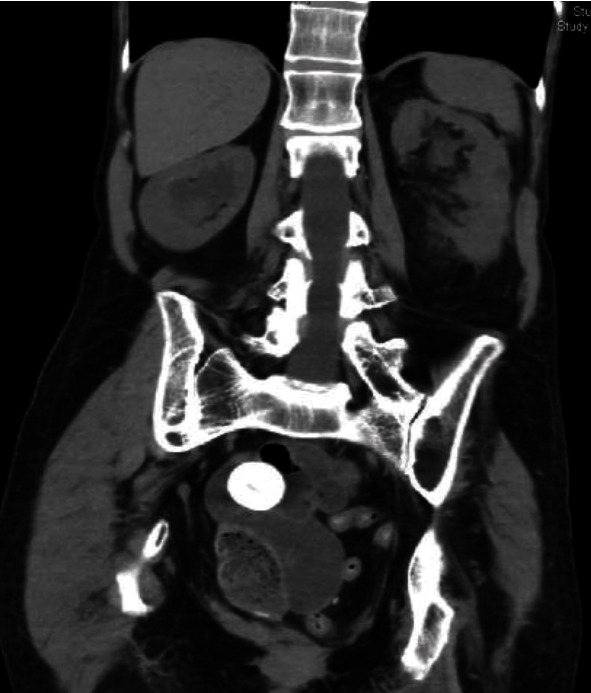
Computed tomography (coronal view) of abdomen and pelvis revealing the 3.1 cm calculus in question.
